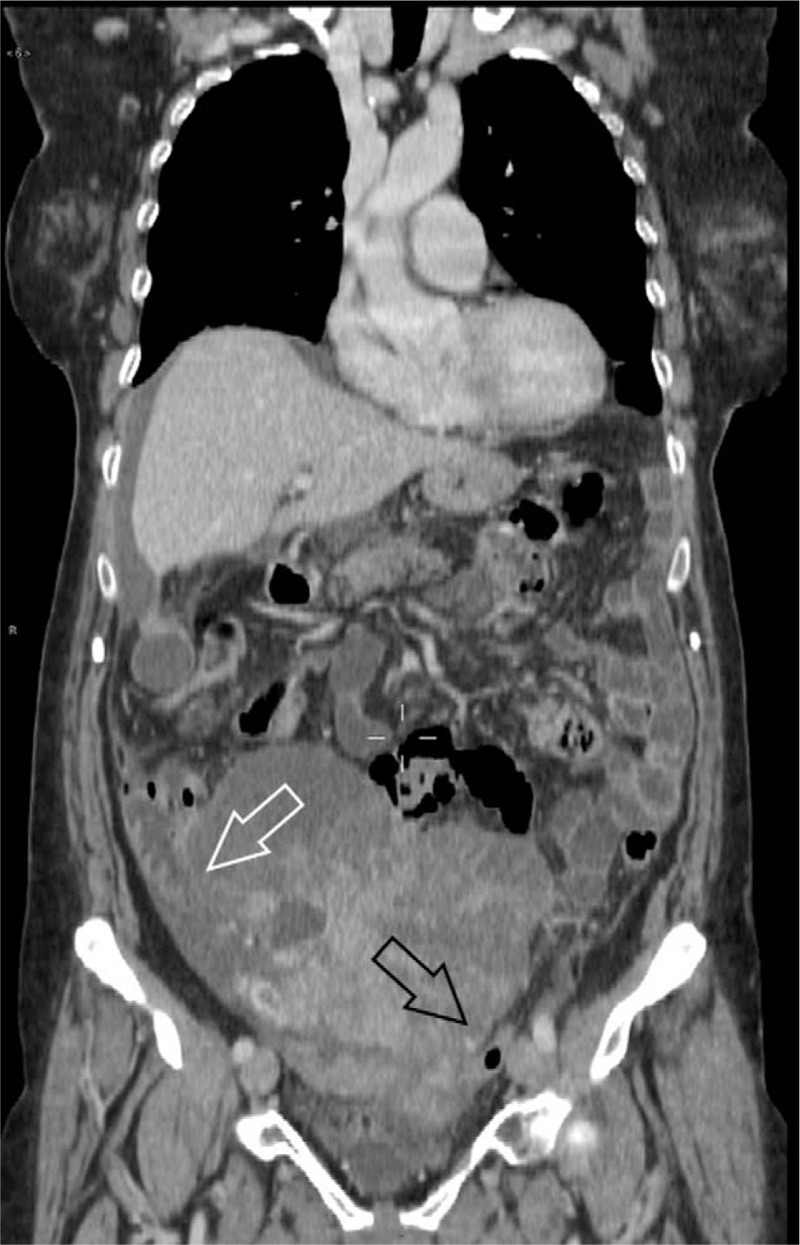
Computed tomography (CT) scan. Inhomogeneous mass tightly attached to the right (white arrow) and sigmoid colon (black arrow).
